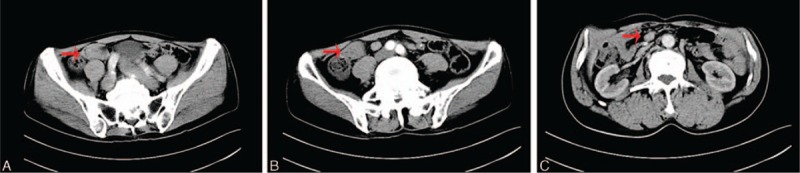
Abdominal CT scan of HAC arising from heterotopic pancreas in the ileum. A, CT scan showed an equal density nodule sized about 2.5 cm × 1.8 cm located on the proximal ileum (indicated by red arrow). B, We found no obvious enhancement in the contrast (indicated by red arrow). C, The edge of the ileum was smooth and clear, and we found no malignant sign, but 1 enlarged lymph node (about 1.7 cm × 1.1 cm) on the mensentery (indicated by red arrow). CT = computed tomography, HAC = hepatoid adenocarcinoma.
